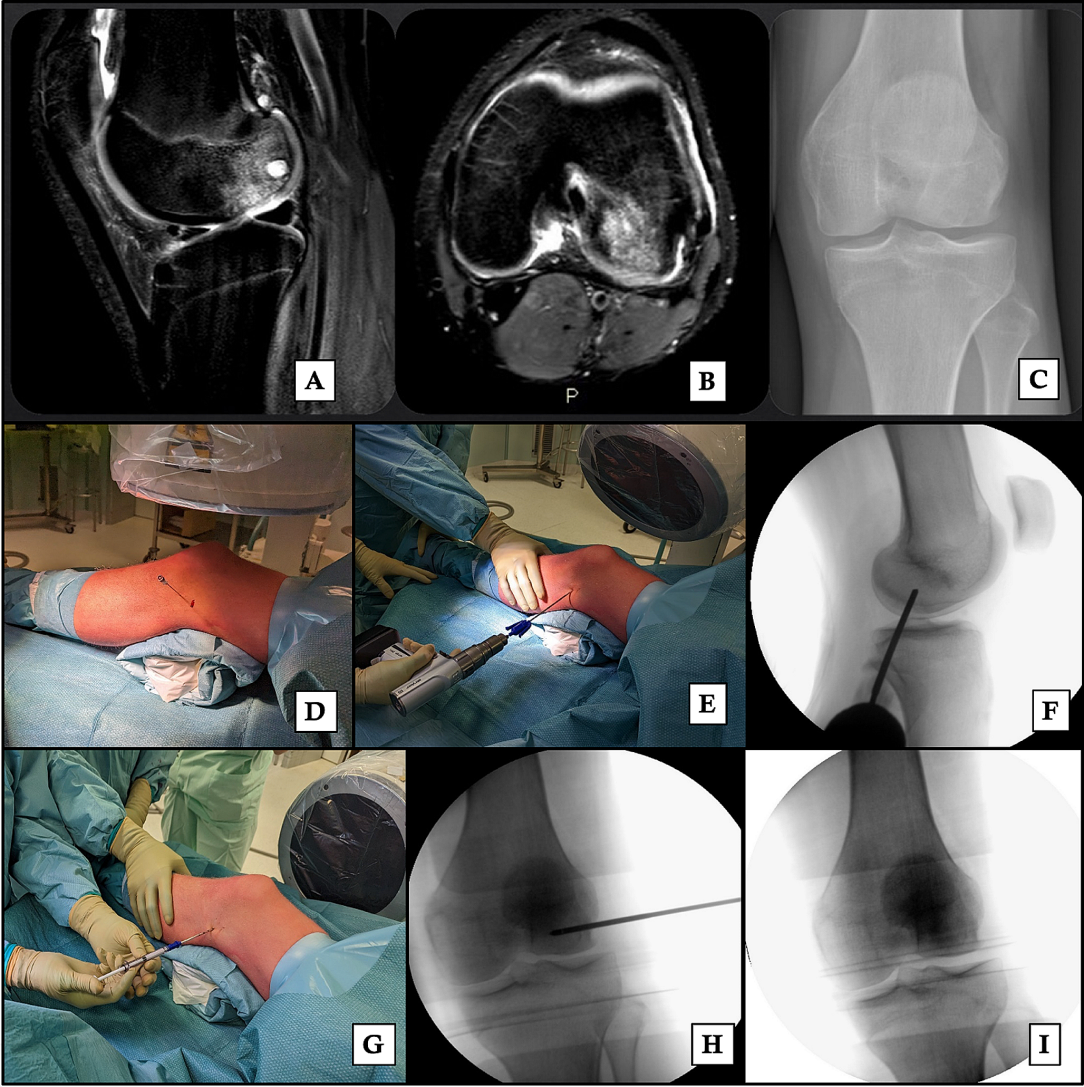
26 years male with a BME of the lateral femoral condyle at MRI (A, B) and X-Ray (C) undergoing subchondroplasty. The guide pin is placed under fluoroscopic guidance (D), the fenestrated cannula is inserted inside the lesion (E) and then controlled under fluoroscopic guidance (F). The syringe, pre-filled with calcium phosphate, is placed (G) and controlled under fluoroscopic guidance (H). The calcium phosphate is then injected (I)
